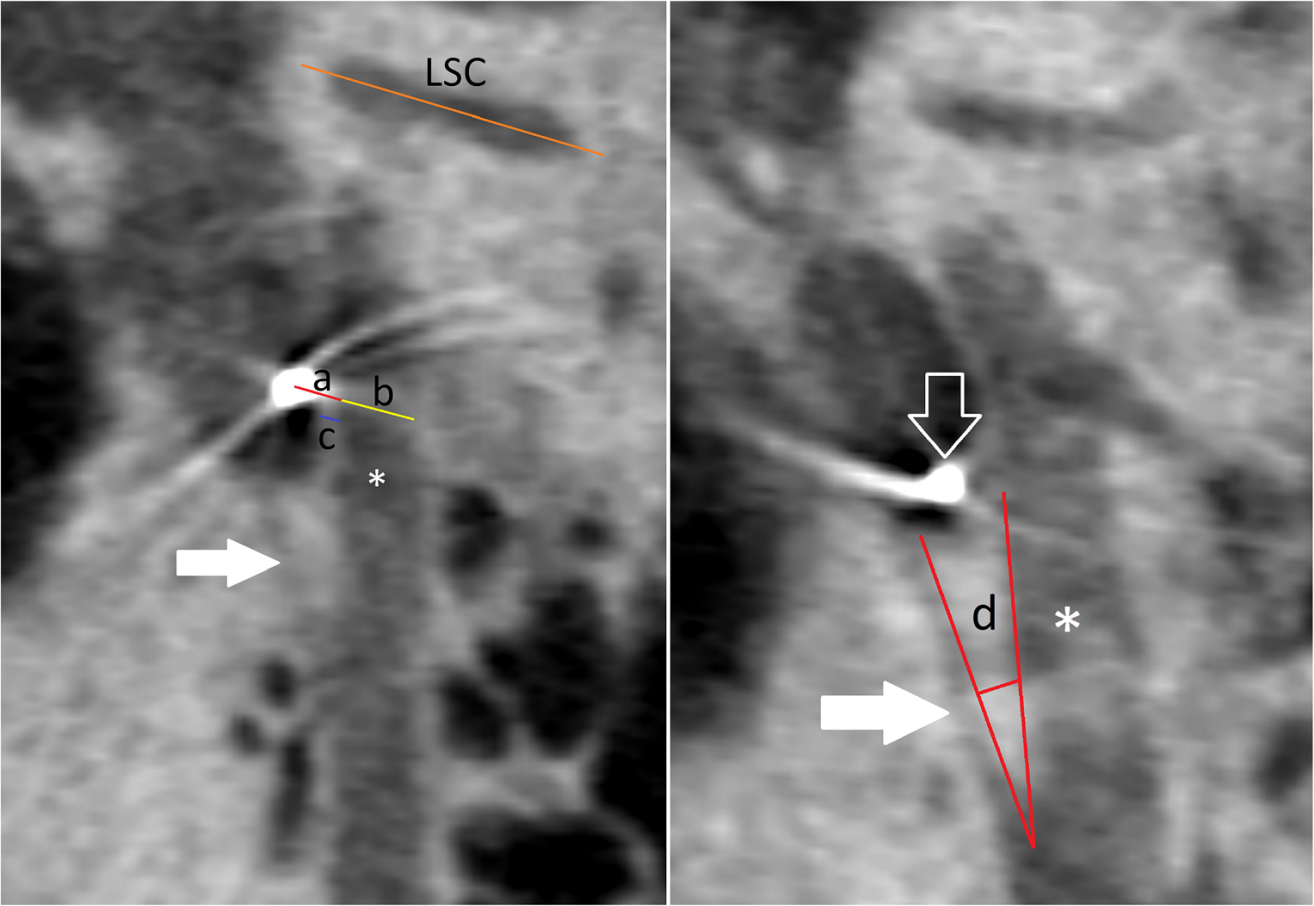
CBCT oblique sagittal reformatted images show measurements of the facial recess region. a: Distance from facial canal (asterisk) to electrode cable (open arrow), b: facial canal diameter, c: facial canal wall thickness, and d: angle between the facial nerve canal and Chorda tympani (white arrow) (LSC, lateral semicircular canal)
